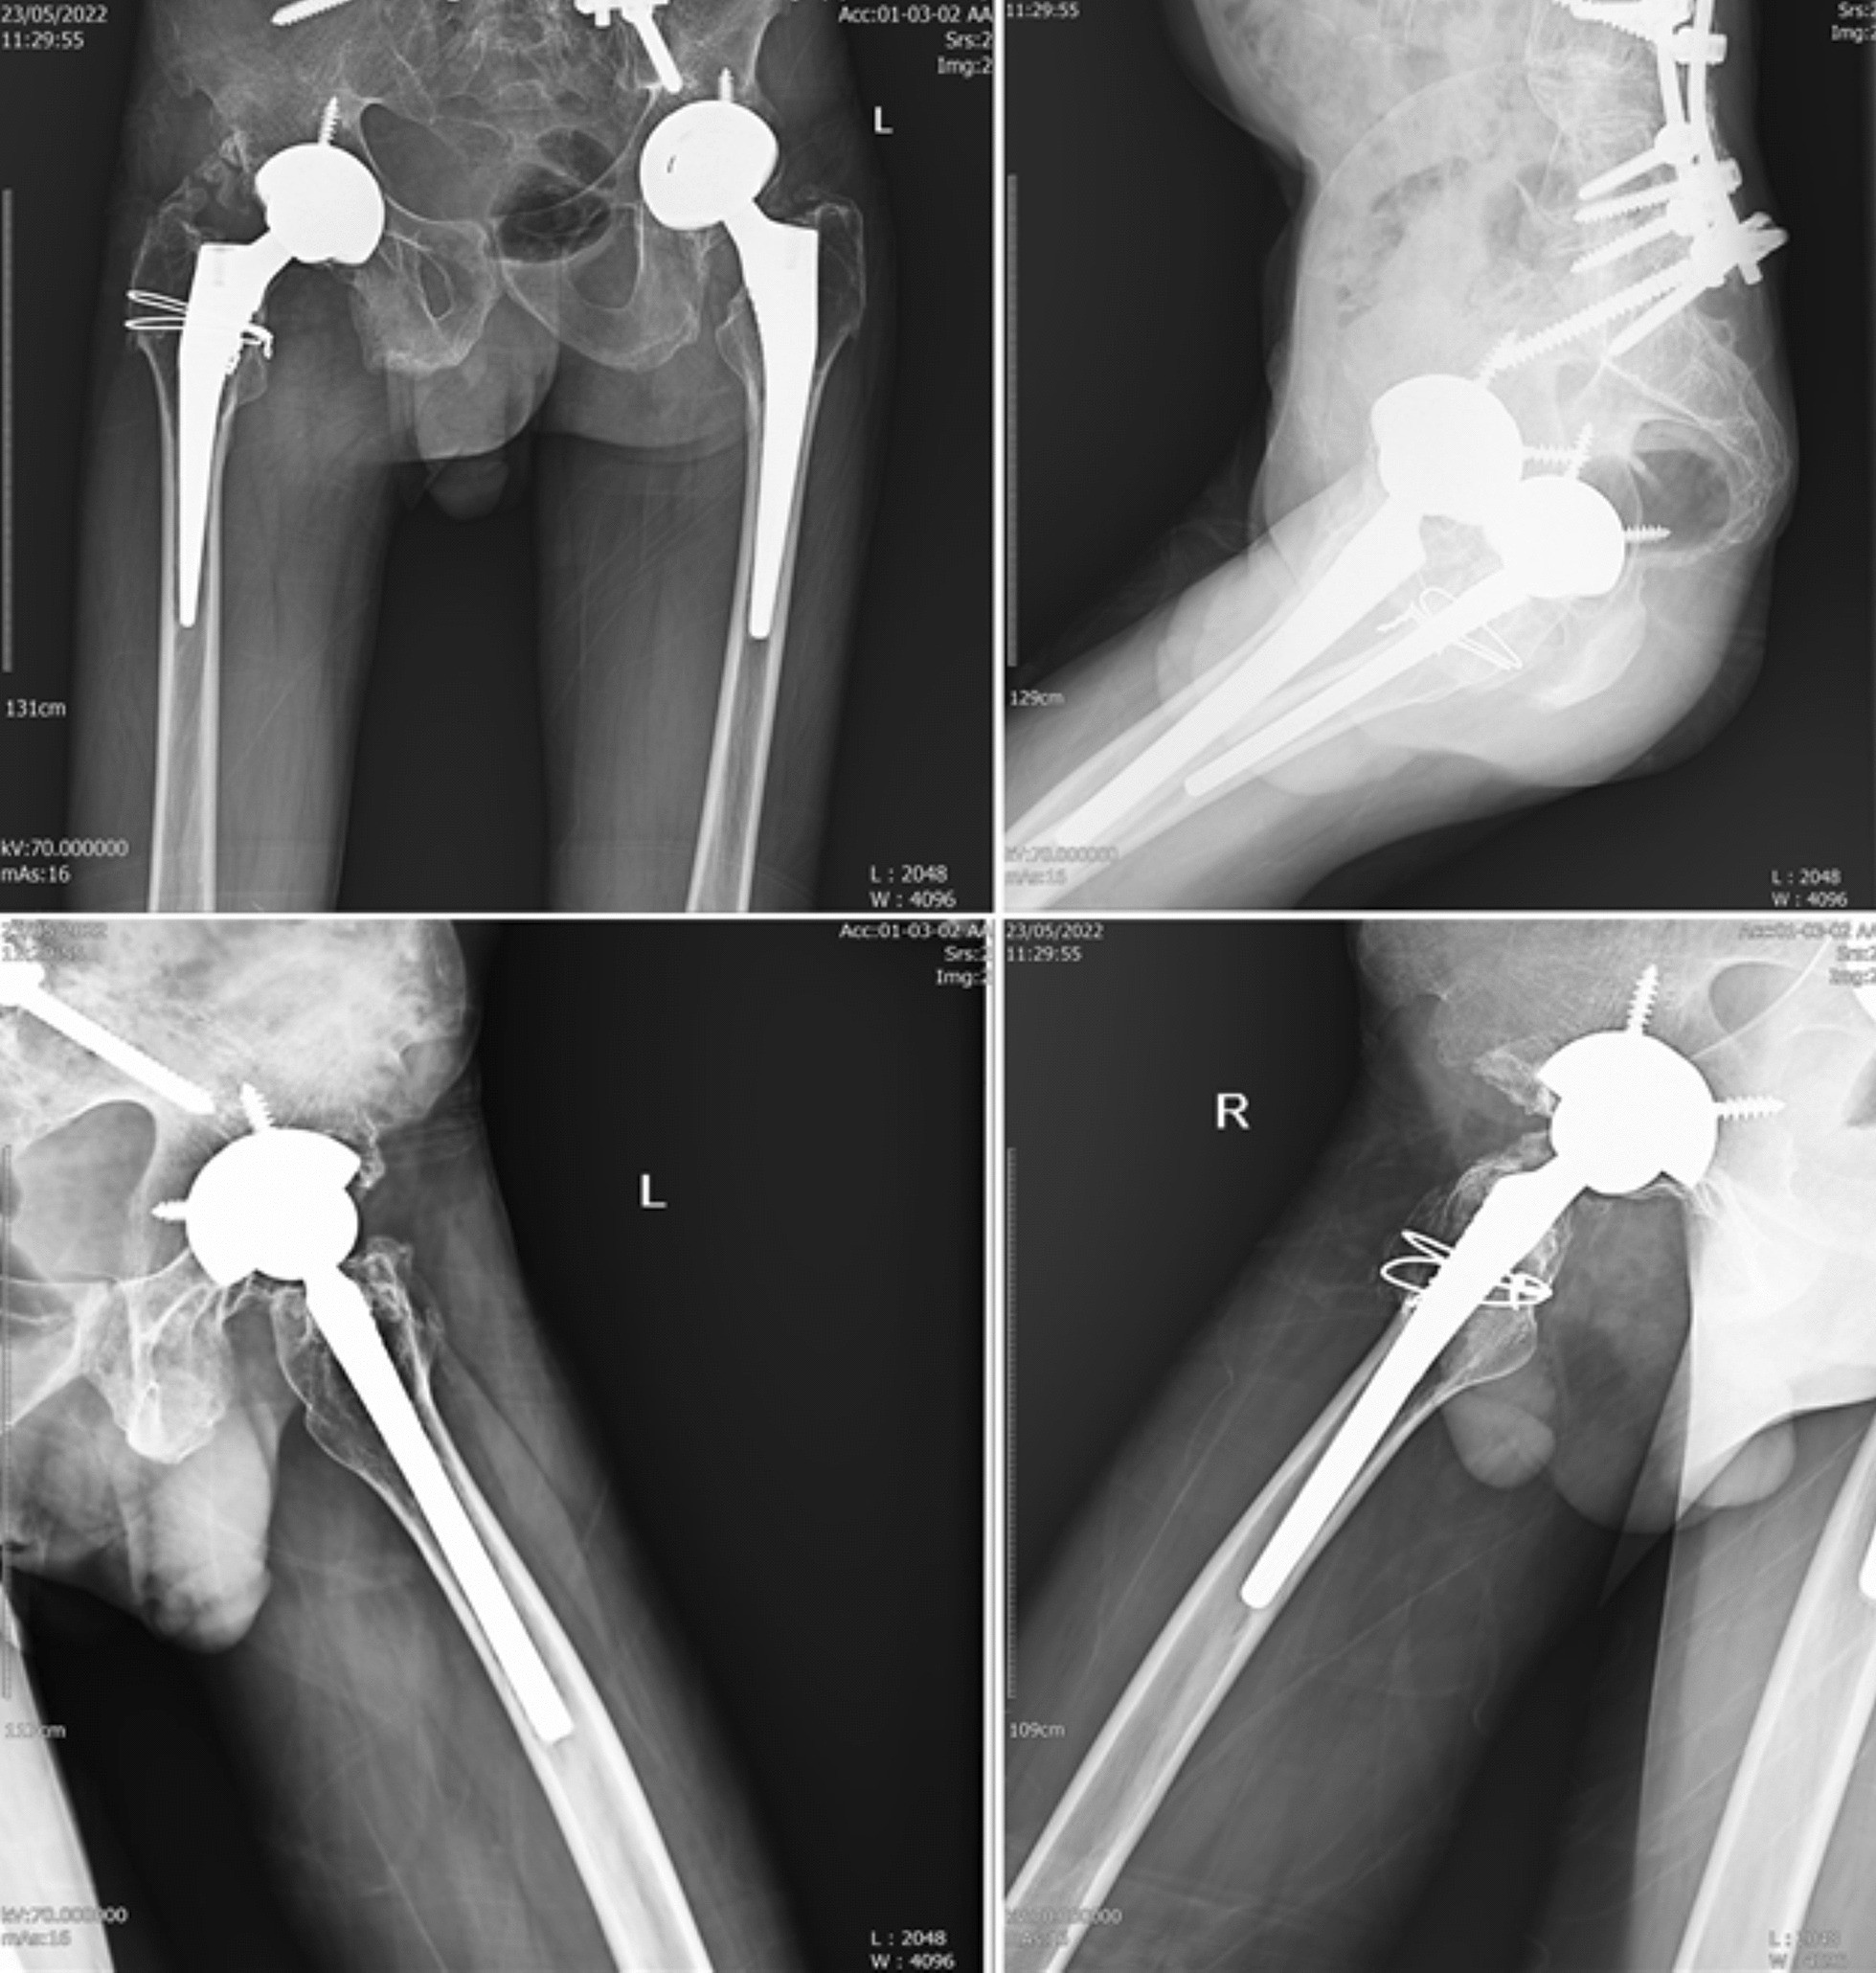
Post-operative plain radiograph revealing the placed arthroplasty and devices
